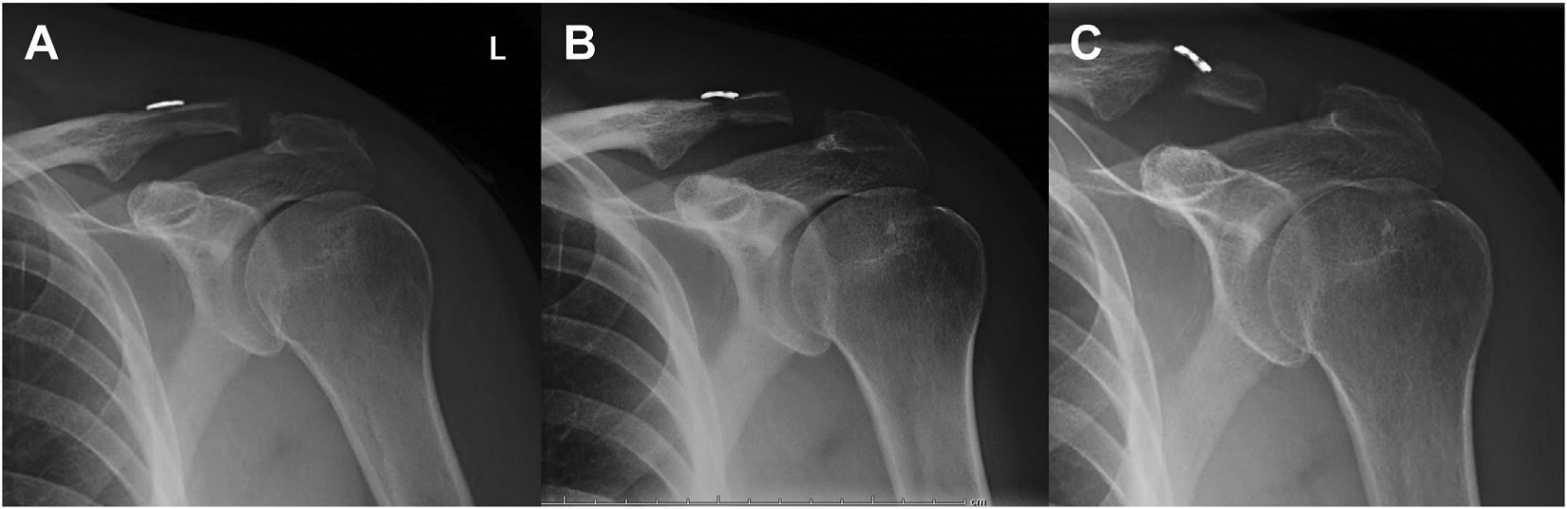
Sequential postoperative radiographs of a right shoulder after acromioclavicular joint reconstruction using a cortical button. Postoperative radiographs are shown at two weeks (A), followed by tunnel widening noted at six weeks (B), and subsequent clavicle fracture at three months (C).
